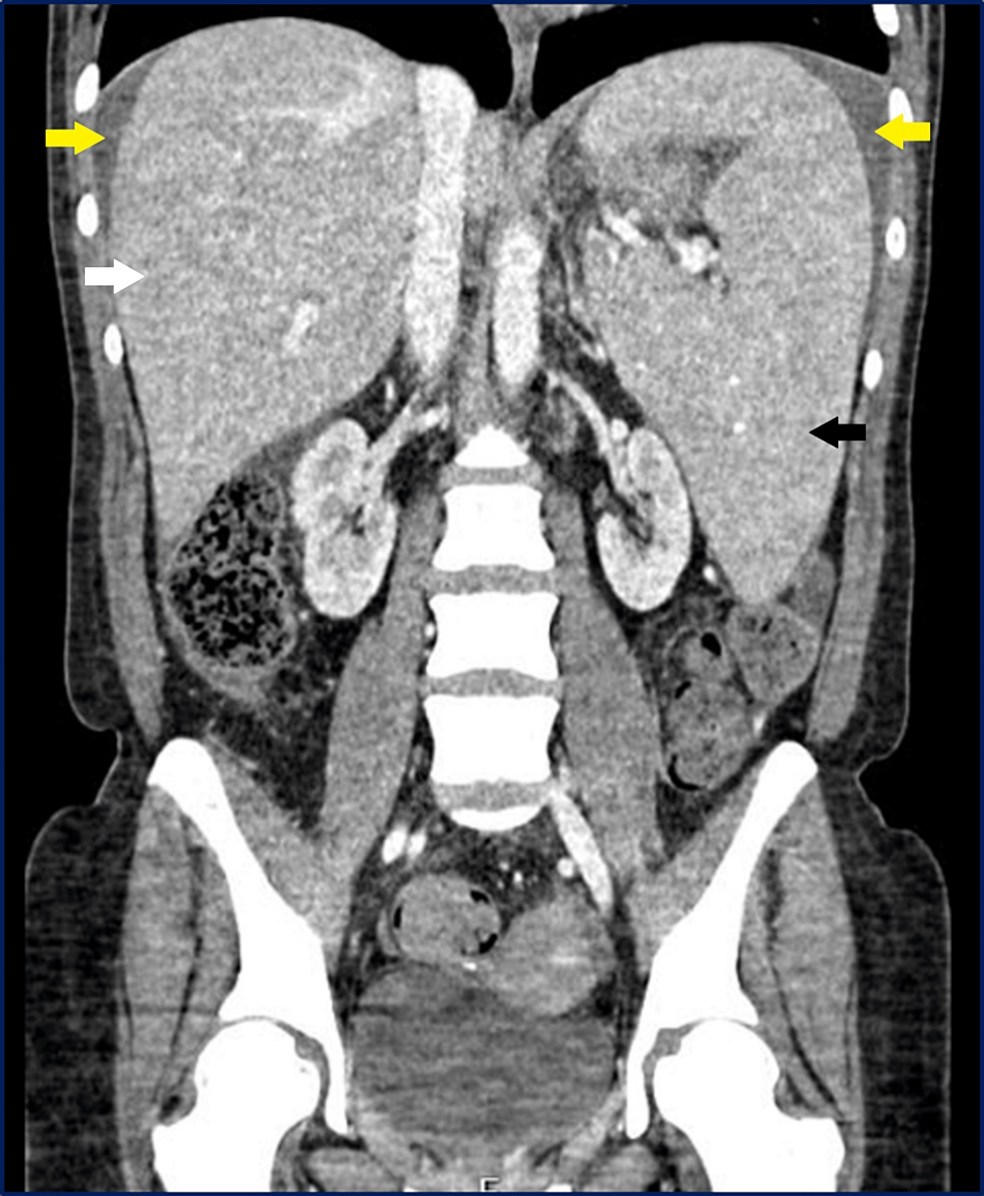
Coronal section of abdominal computed tomography showing hepatomegaly (white arrow), splenomegaly (black arrow), and free peritoneal effusion (yellow arrow).
